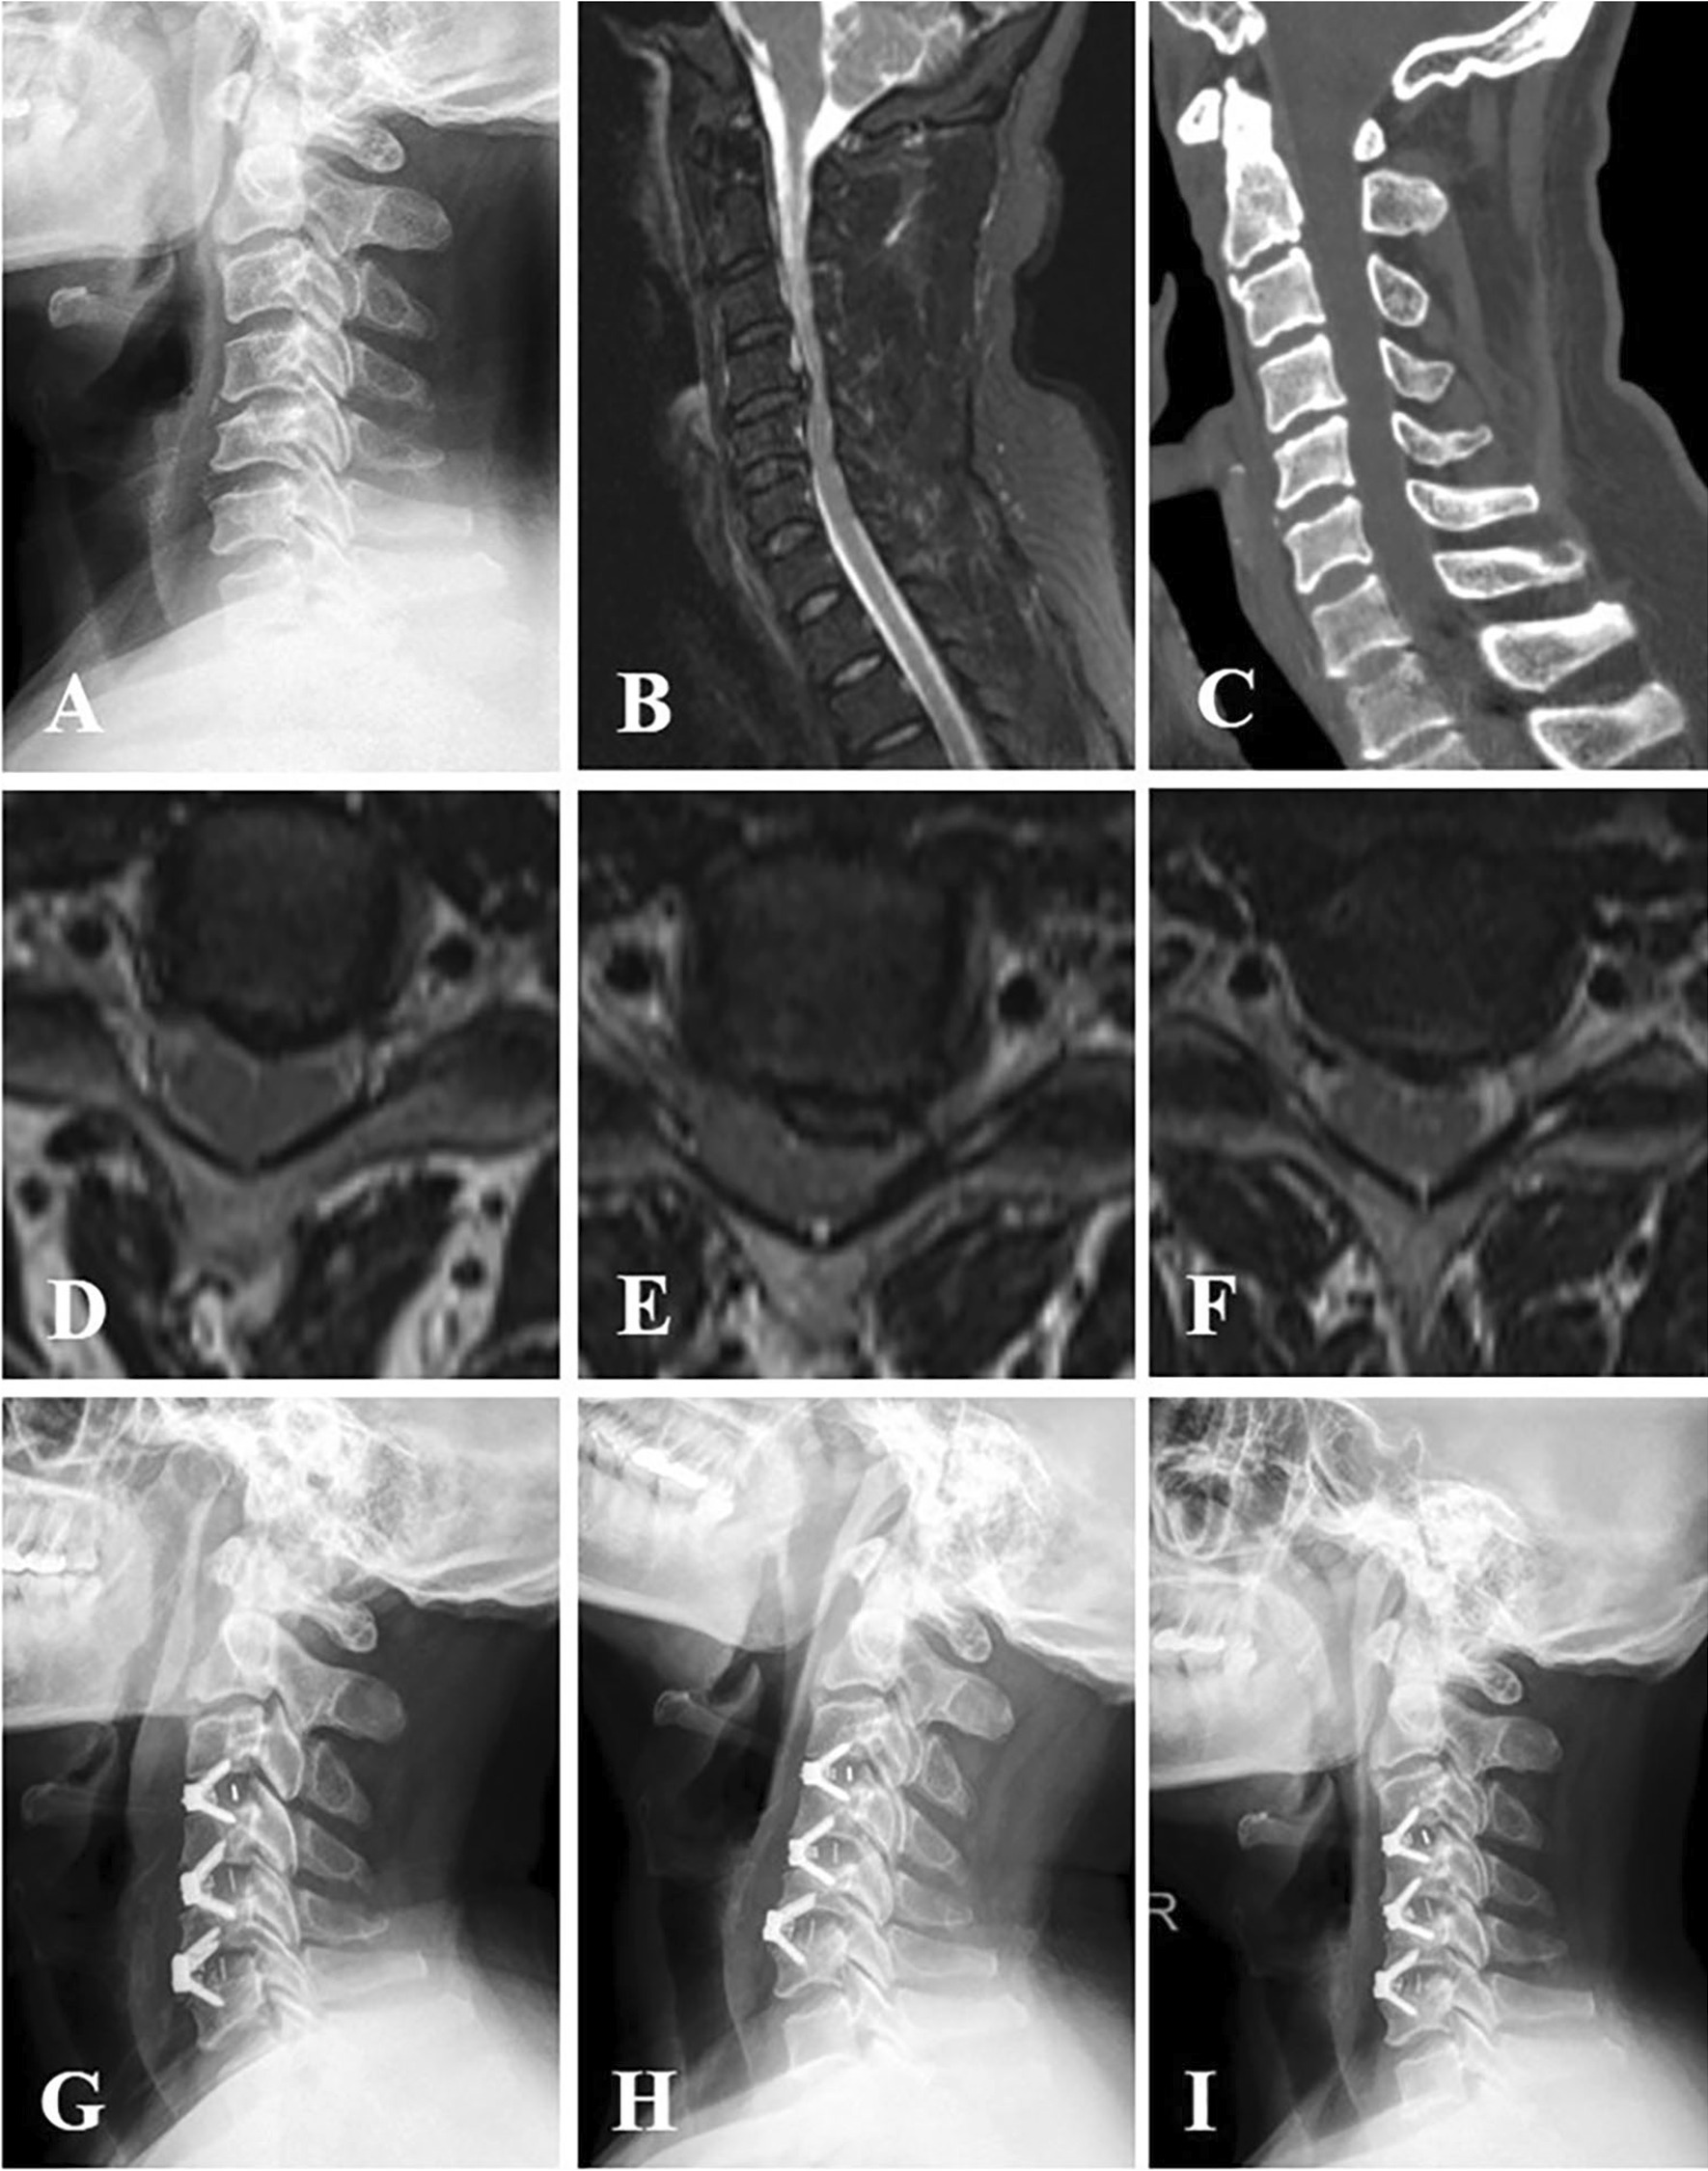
A 53-year-old man who had experienced numbness in both hands and felt stepping on cotton in lower limbs for 1.5 years was diagnosed as cervical spondylotic myelopathy. A Preoperative cervical lateral X-ray plain radiograph showed the alignment of cervical spine was mild kyphosis. B, D, E, F Cross-sectional and sagittal T2-weighted MRI showed degenerative disc protrusion and spinal cord compression at C3–C4, C4–C5 and C5–C6, respectively. C Sagittal CT reconstruction showed that no osteophyte compression was found anterior to the spinal canal. The patient who underwent three-level ACDF with stand-alone self-locking cages. G The X-ray examination showed the alignment of cervical spine improved significantly two days postoperatively. H X-ray film which was taken six months postoperatively identified that the alignment of cervical spine was well maintained, and the positions of cages were good. I At twelve-month follow-up, the positions of cages were still remained, whereas the lordosis of cervical spine is reduced
